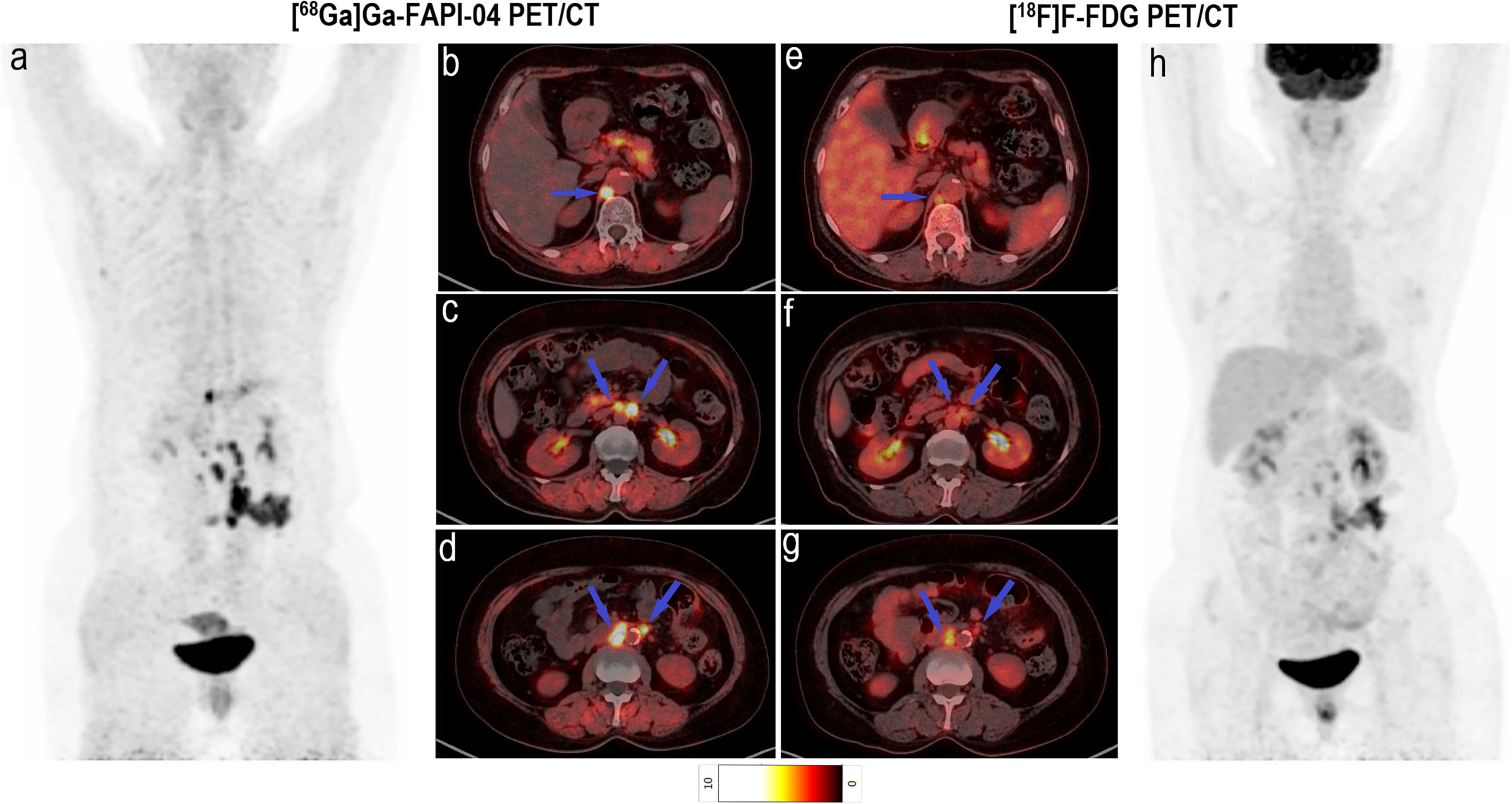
Images of a 64-year-old woman with colon cancer for staging. (A) Mean intensity projection images of [^68^Ga]Ga-FAPI-04 PET/CT scans. (B–D) Axial fused images of suspicious lymph nodes at different levels of the body in [^68^Ga]Ga-FAPI-04 PET/CT. (E–G) Axial fused images of suspicious lymph nodes at different levels of the body in [^18^F]F-FDG PET/CT. (H) Mean intensity projection images of [^18^F]F-FDG PET/CT scan. [^68^Ga]Ga-FAPI-04 uptake (blue arrows in B–D) was higher than [^18^F]F-FDG uptake (blue arrows in E–G) in both the diaphragmatic and retroperitoneal metastatic lymph nodes, with SUV_max_ values of 5.9–12.0 and 3.4–4.3, respectively.
